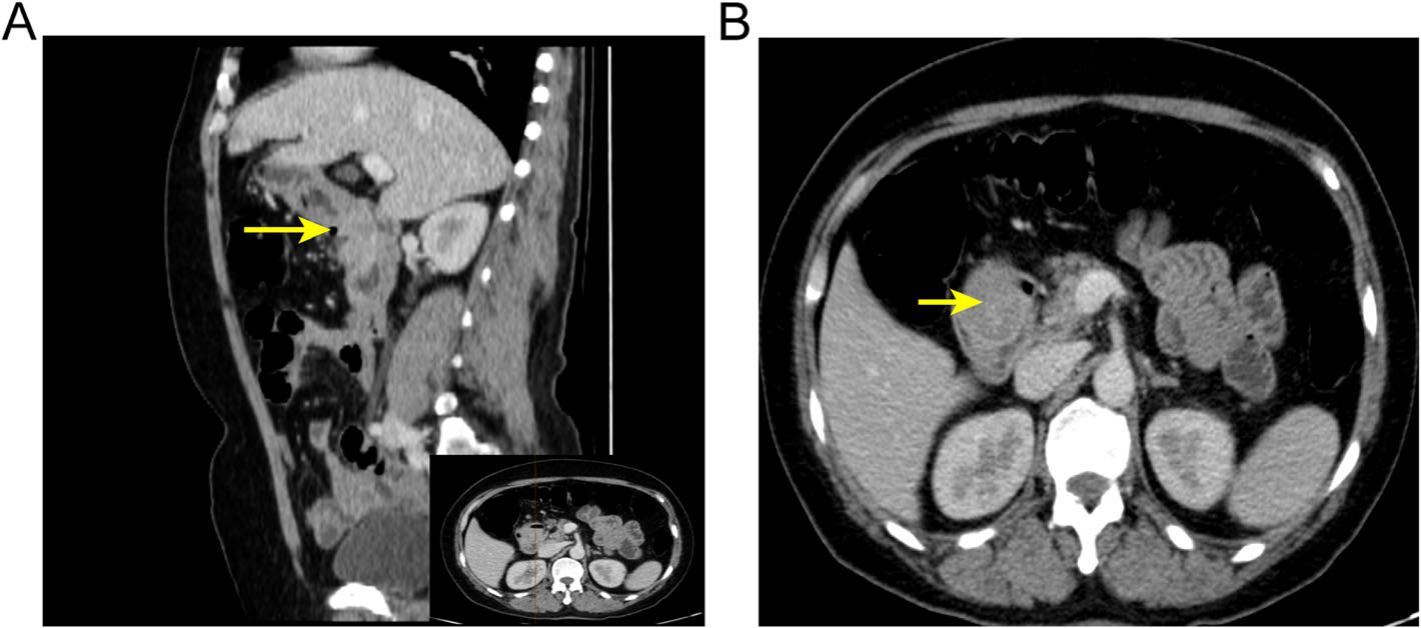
(a) Contrast-enhanced sagittal and (b) axial computed tomography scan shows a large polypoid mass in the duodenal bulb (arrows)
